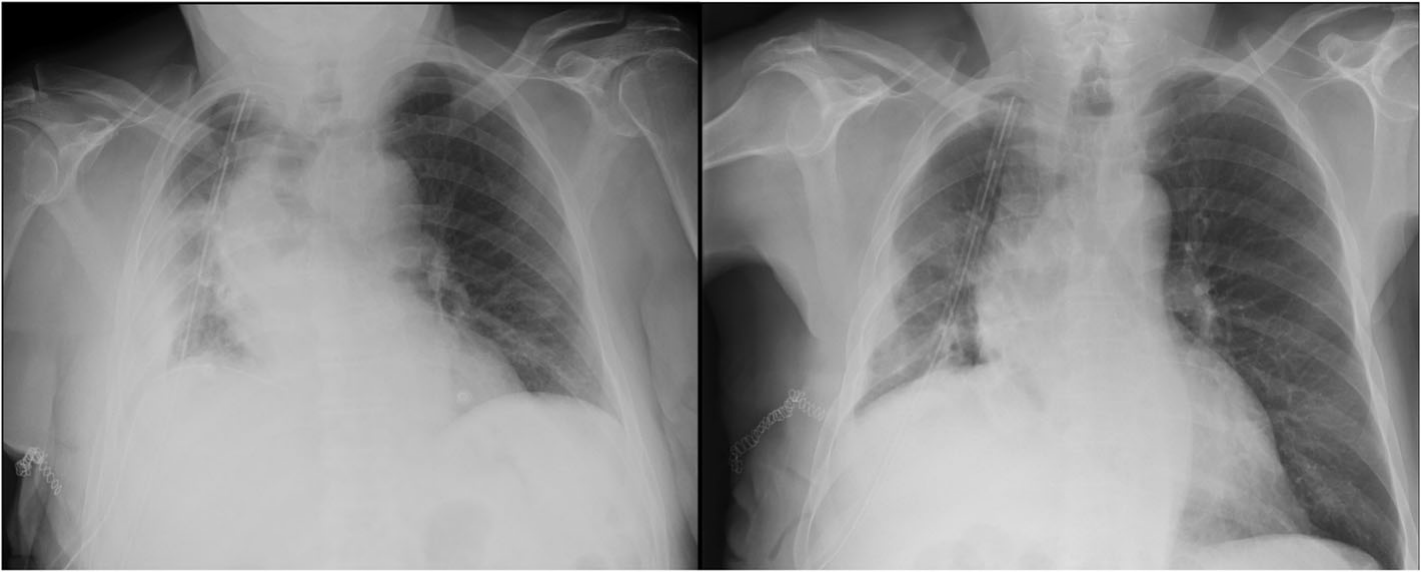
Chest x-ray performed before (on the right) and after (on the left) bronchoscopy. Chest x-ray reported the presence of loculated pleural effusion, which was correctly identified as obstructive atelectasis by Chest US (see Fig. 4)
